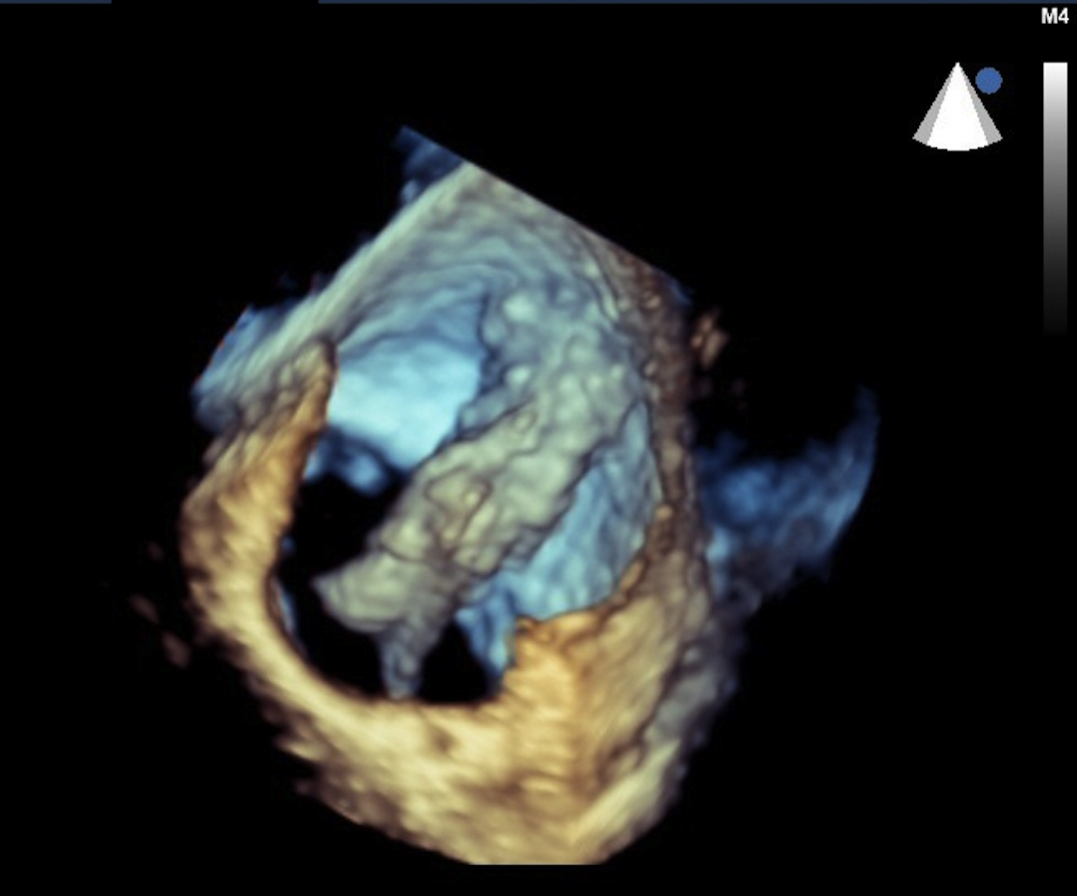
3D transesophageal echocardiogram shows a large left atrial mass and its attachment point on the posterior left atrial wall.
